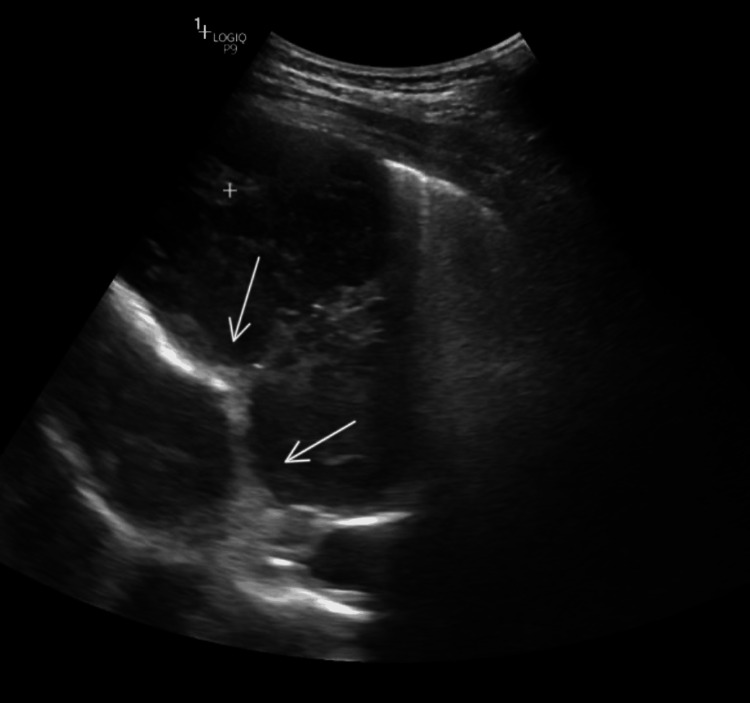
Ultrasonogram showing solid lesion with internal septations in the left lower lobe (arrows)
